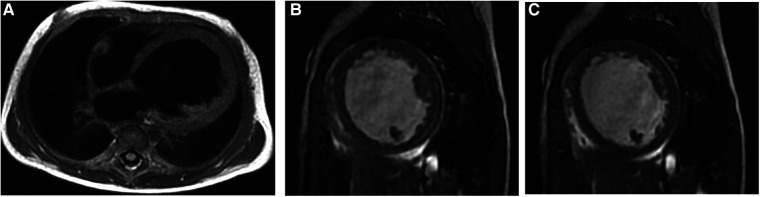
Representative MRI scans of a 5-month female EFE patient who presented with cyanosis and shortness of breath. (A) Four-chamber D-IR FSE showing LV globular enlargement; the ventricular septum is obviously protruding to the right ventricular side. (B,C) Short-axis FIESTA cine at the end of systole and diastole, respectively, showing thickened endocardium and reduced movement of the LV. EFE, endocardial fibroelastosis; LV, left ventricle.
